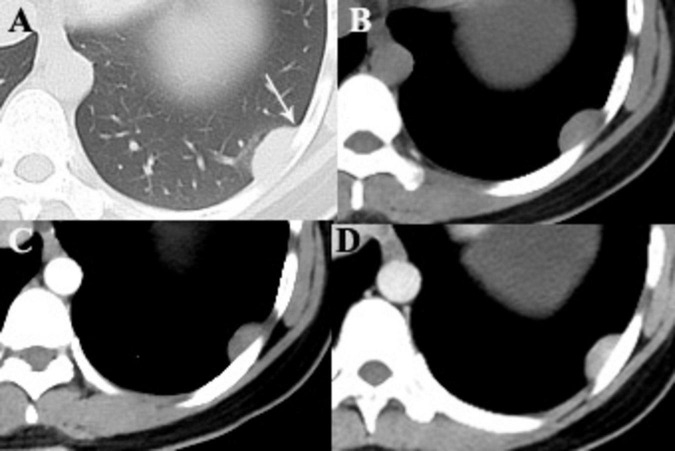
A 28-year-old woman with SFTP. (A,B) Precontrast CT scans demonstrate a small well-defined mass with homogeneous density in the lower left thorax. The mass forms an obtuse angle with the adjacent pleural surface (arrow). (C) No intratumoral vessels are detected in the arterial phase on postcontrast CT image. (D) Obvious enhancement is seen in the venous phase.
